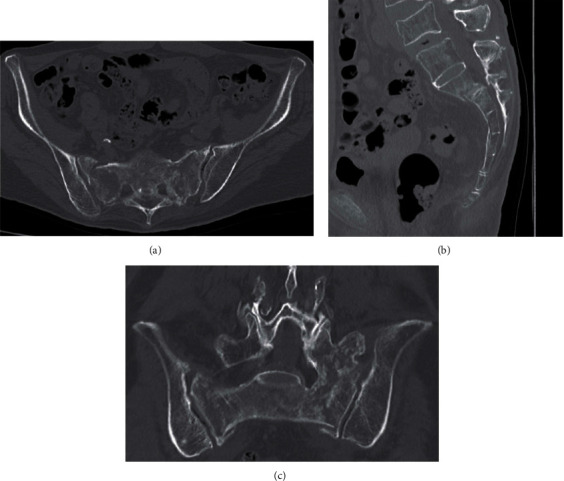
CT ((a) axial, (b) sagittal, and (c) coronal) showing displaced bilateral sacral alar fractures. L5 was bonny fused with S1.
